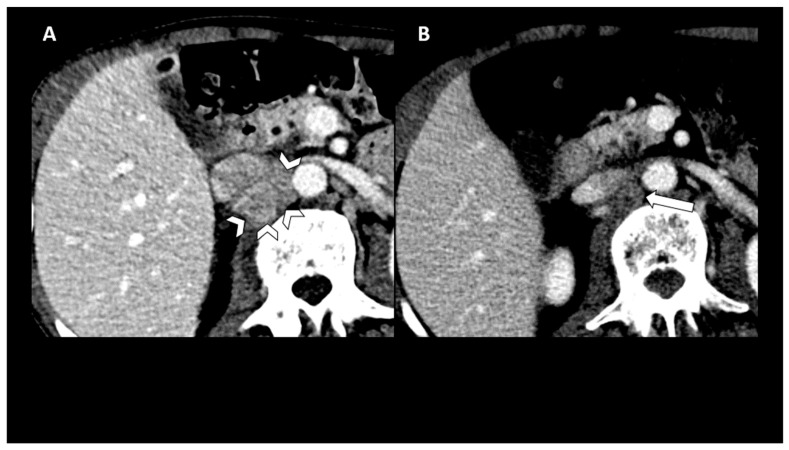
CT scan images from baseline (A) and post treatment (B). Time points represent significant decrease in size and enhancement in confluent retrocaval and aortocaval lymphadenopathy (arrowheads) with mass effect on the inferior vena cava (IVC) at baseline.
